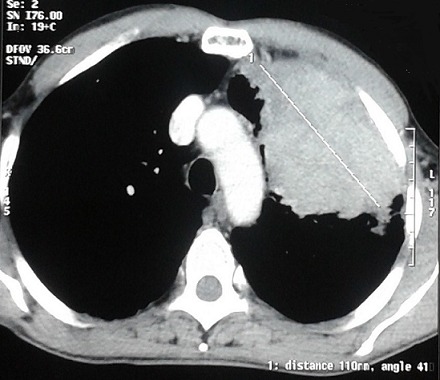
CT scan of the chest showing a tumor mass in the left upper lobe which measures 90 × 76mm
